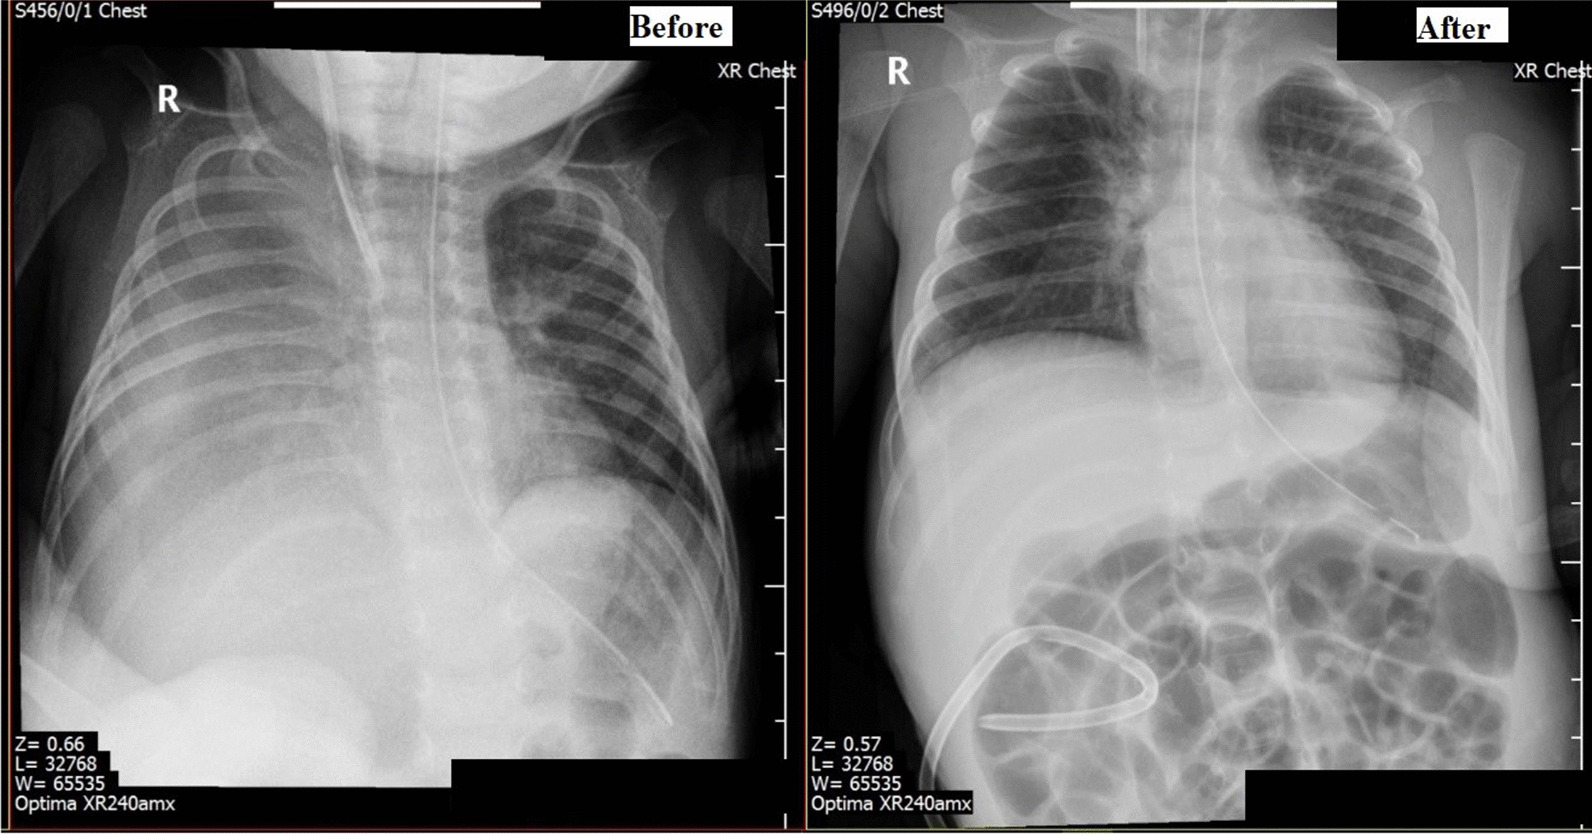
Chest X-ray showing before and after perinephric insertion with right-sided pleural effusion improvement
